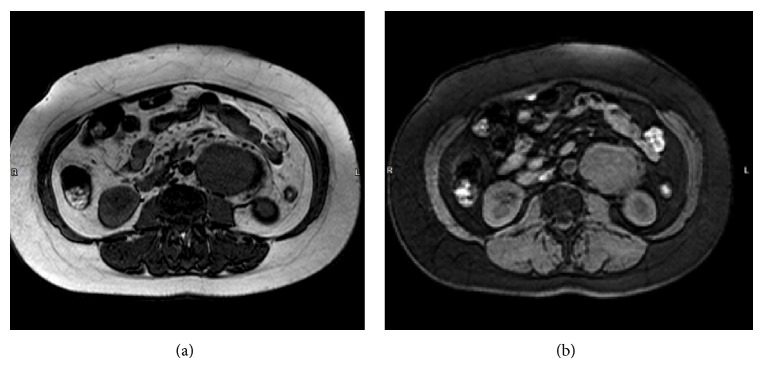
(a), (b) Preoperative magnetic resonance imaging (MRI). A left pararenal mass is shown (T1 and T2 hyperintensity, resp.).
